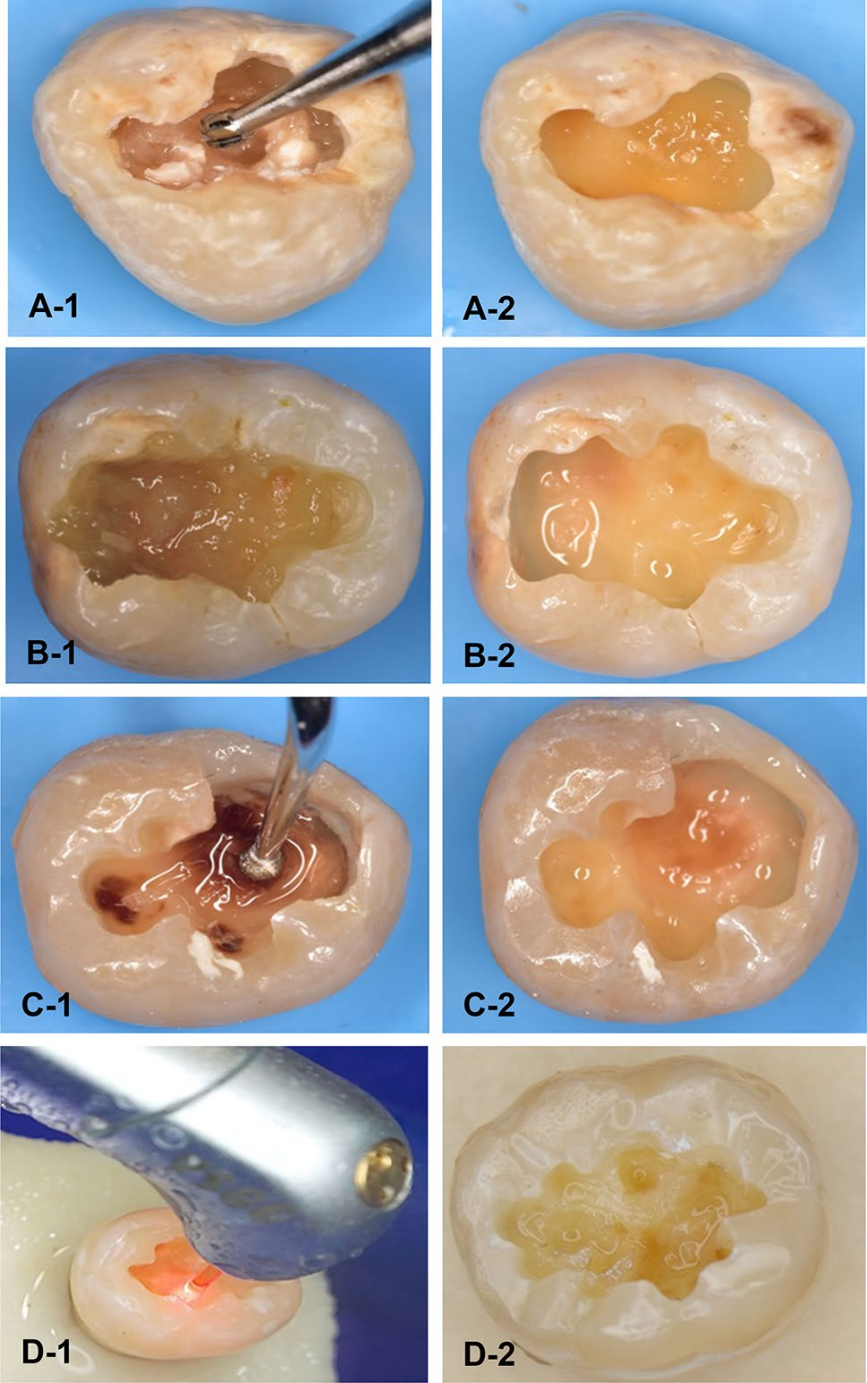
The caries excavation techniques for removing deep carious dentin lesions. (A-1) rotary excavation, (B-1) Brix 3000 application, (C-1) Ultrasonic Caries Removal Tip SB1, (D-1) Waterlase iPlus Er,Cr:YSGG Laser. (A-2,B-2,C-2,D-2) The residual dentin after each technique, respectively.
